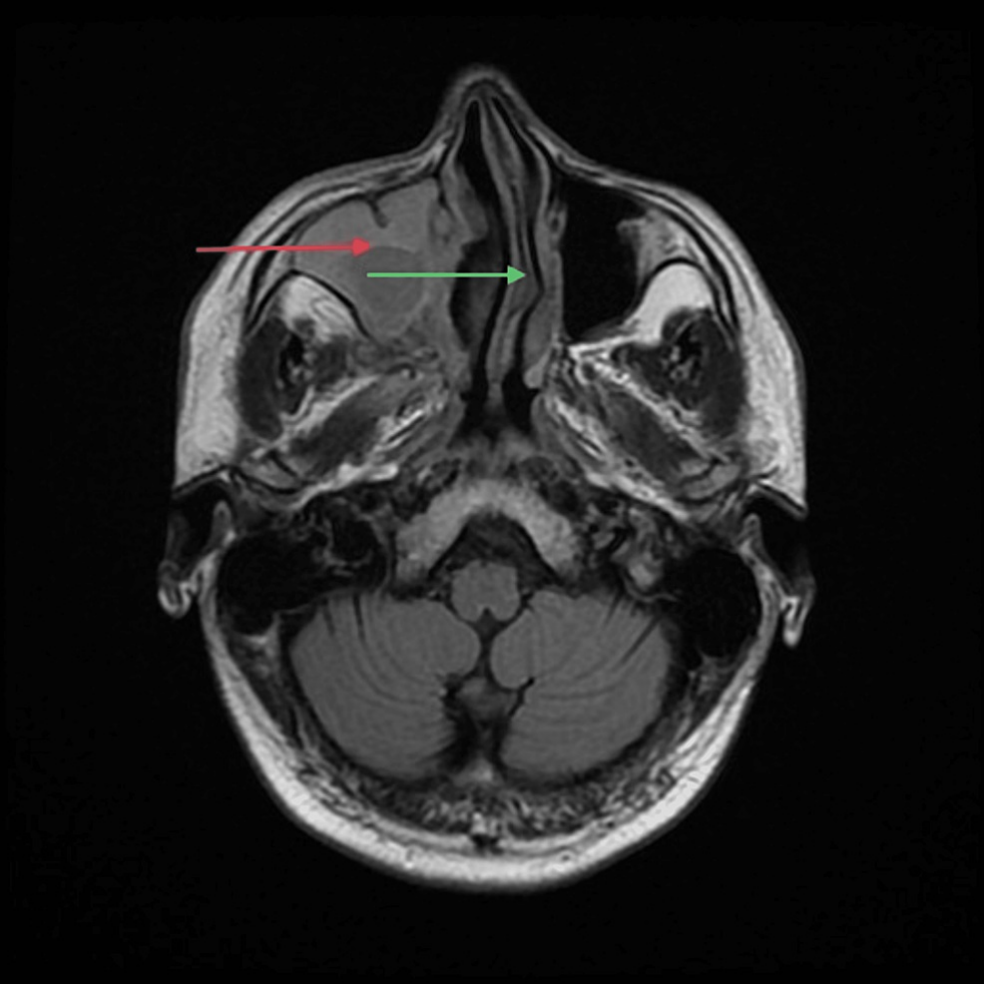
T1-weighted MRI head (axial section) of Case Five showing right maxillary sinusitis and infiltration (red arrow), and nasal septum deviation (green arrow)
